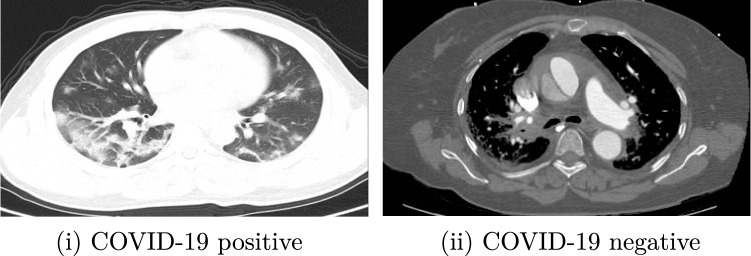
Sample images from COVID-19 CT Scan dataset belonging to corresponding classes
